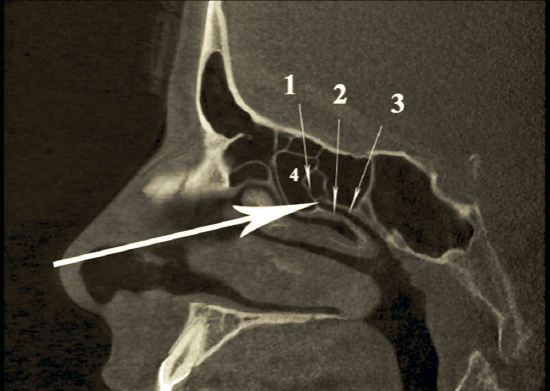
Sagittal CT demonstrating the surgical strategy to open the posterior ethmoid. After opening the basal lamella of the middle turbinate (1) directly above the horizontal part (2), the superior meatus (3) is reached. (4) = ethmoid bulla.
